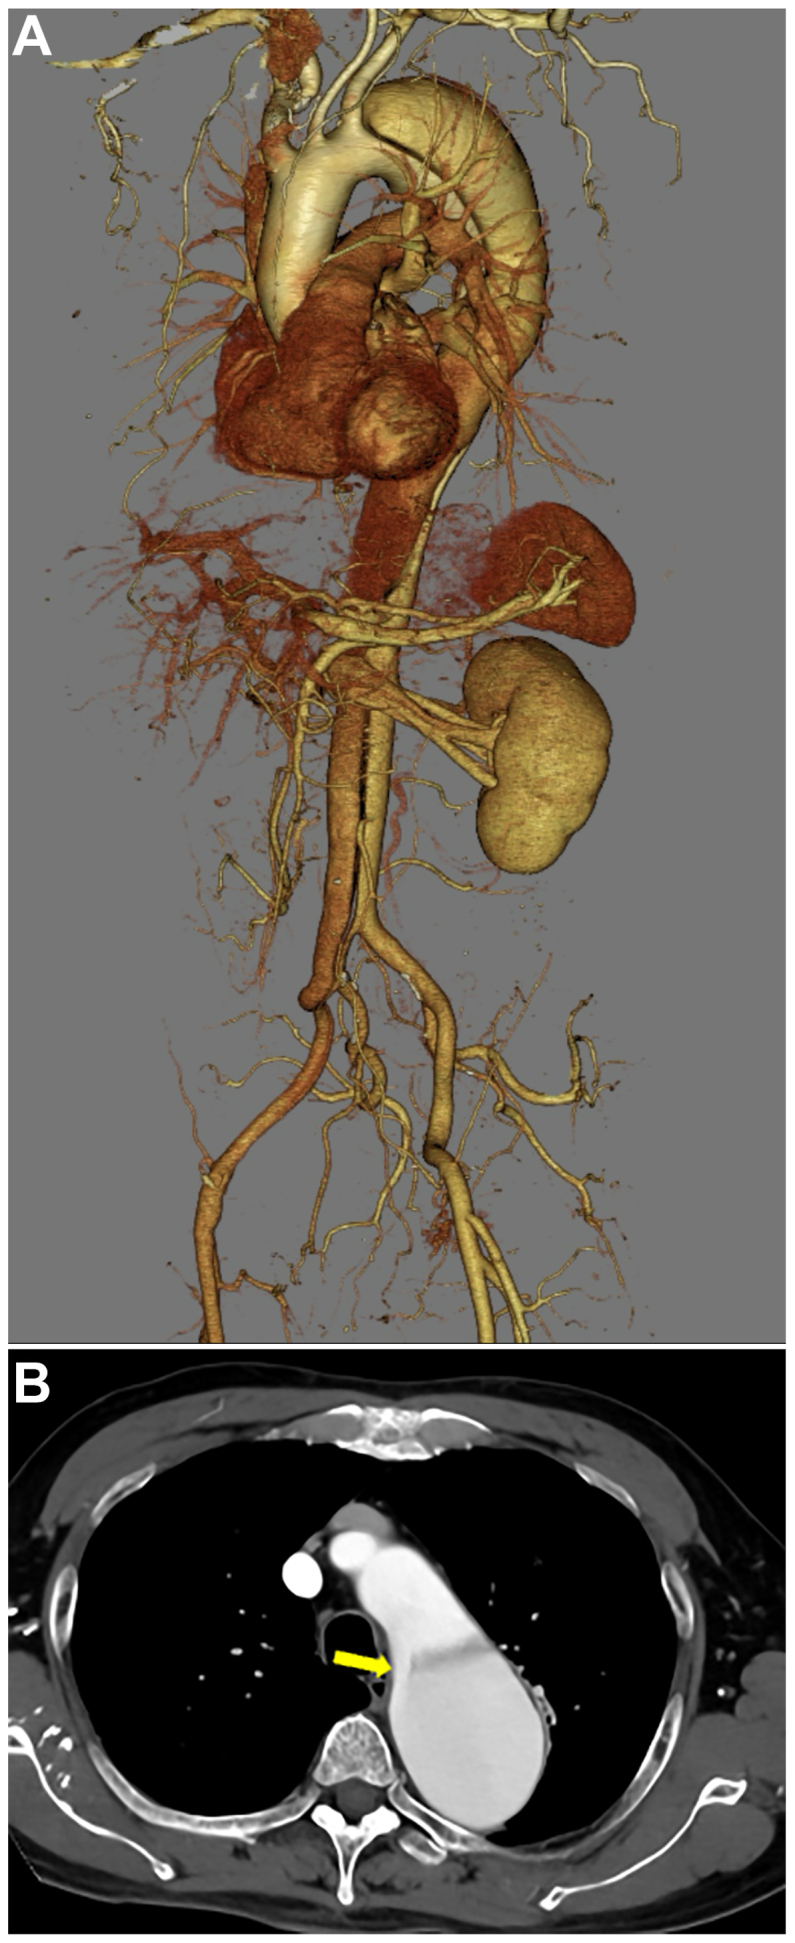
Volume rendering of computed tomography (CT) angiography demonstrates a chronic type B aortic dissection (TBAD) extending from just distal to the left subclavian artery (LSA) to the right external iliac artery (A). Axial CT image shows the primary entry tear (*arrow*) in the distal aortic arch (B).
